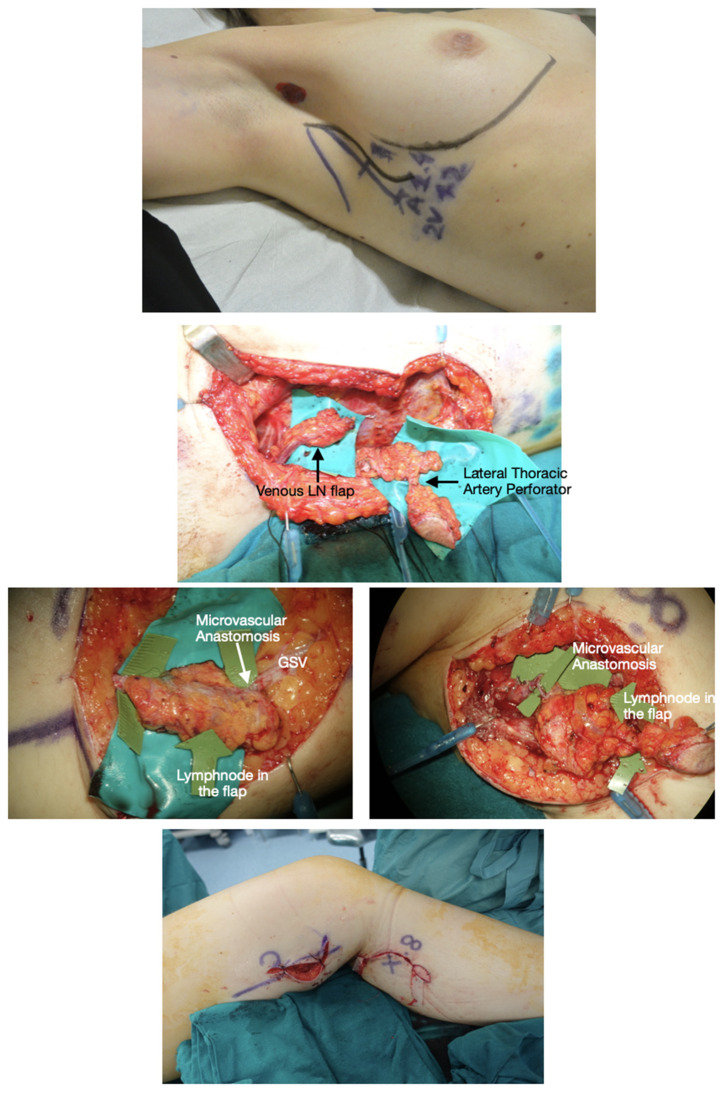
Lateral thoracic VLNT (LT-VLNT). Above: Preoperative planning of LT-VLNT using the 18-MHz linear probe and ICG-lymphography of the lateral thoracic region. Notice the black dot in the axilla showing the node marked by reverse mapping using lymphoscintigraphy. The incision is planned not to alter the breast aesthetic as a vertical lazy-S. The inframammary fold is marked to avoid any violation of this important aesthetic structure. Second row: Dual LT-VLNT has been harvested. The uppermost flap is a venous lymph node flap based on the thoracoepigastric vein. The lower VLNT flap, including a monitor skin paddle based on a perforator marked preoperatively, is based on the lateral thoracic artery, comintantes vein and thoracoepigastric vein lower portion. Third row, left: The venous VLNT is anastomosed in a flow-through fashion to the great saphenous vein above the knee. The green arrows show lymph nodes in the flap. Third row, right: The arterovenous VLNT is anastomosed to medial sural vessels in the calf area. Below: Final inset at the end of surgery. The venous flap is buried and monitored in the postoperative time with ultrasound, and the arterovenous flap has a small skin-paddle used for monitoring.
